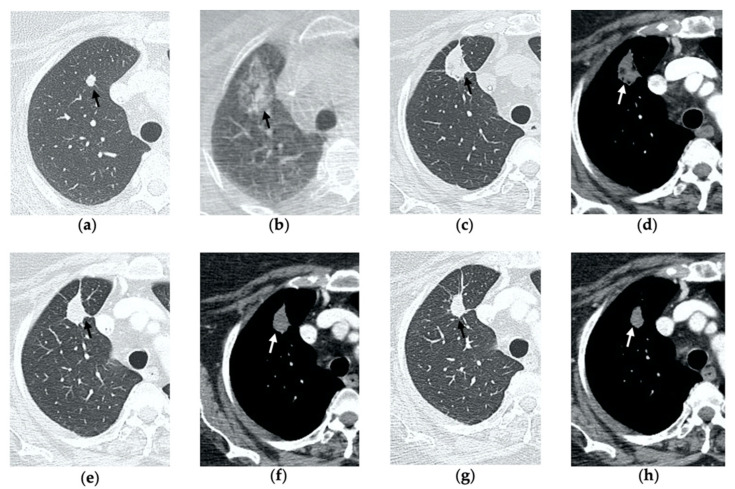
63-year-old woman with pulmonary metastasis from colorectal cancer (CRC) in the right upper lobe. (a) Axial CT of the metastasis (black arrow) prior to treatment. (b) Cone-beam CT image obtained post-procedure shows hazy GGO (black arrow) of the ablation site surrounding the treated nodule. (c,d) Axial 2-month follow-up CT images show a large consolidation with hypoattenuating bubbles (black arrow) and no contrast uptake (white arrow). (e,f) Axial 5-month follow-up CT images demonstrate a decrease in the size of the consolidation (black arrow) with no central contrast enhancement (white arrow). (g,h) Axial CT images obtained after 8 months show minimal further decrease in size with no signs of residual disease.
